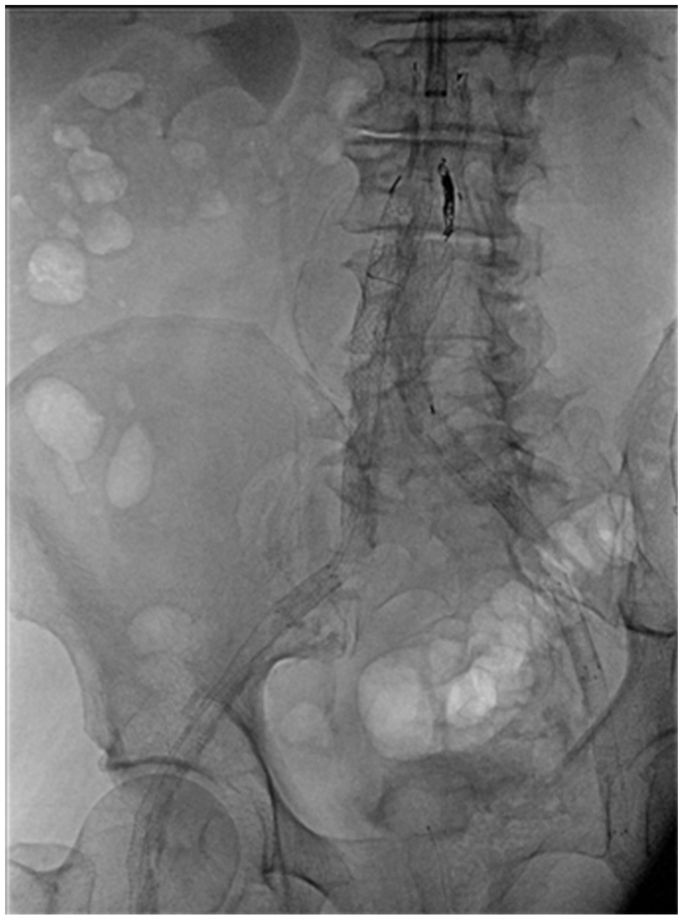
Plain radiograph revealing final graft configuration of the Gore Excluder Endograft to exclude the abdominal aortic aneurysm (AAA).
